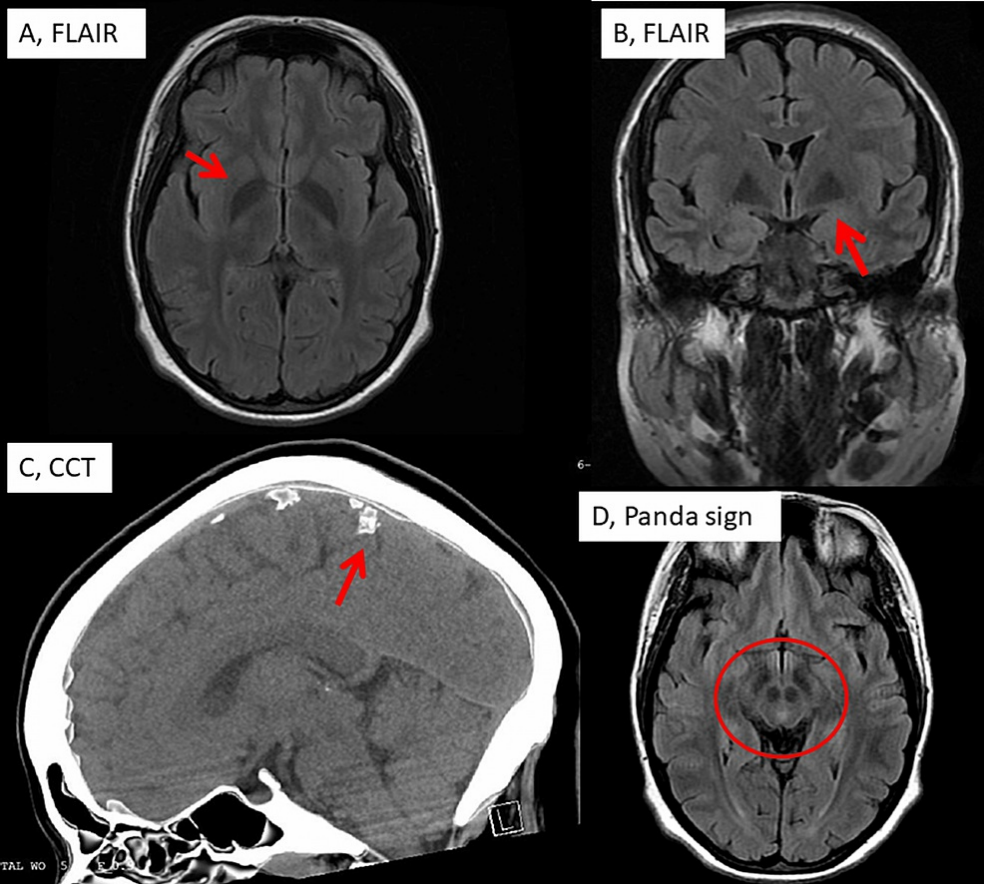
Magnetic resonance imaging (axial and coronary FLAIR images) showing hypointensity of the globus pallidus bilaterally (A, B). FLAIR images of the midbrain showing a distinct Panda sign (D). Cerebral CT of the brain (sagittal plane) showing abnormal calcifications in the subarachnoid space CCT: cerebral CT, FLAIR: fluid attenuated inversion recovery
